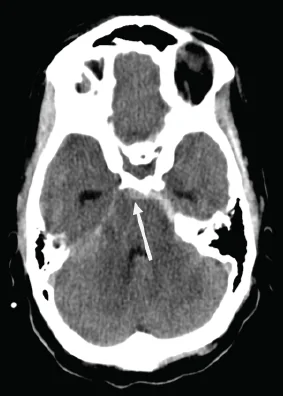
Computed tomography of the head (axial view) at the level of the foramen magnum within the prepontine cistern showing hemorrhage into the spinal canal (arrow) in a patient diagnosed with perimesencephalic nonaneurysmal subarachnoid hemorrhage.
